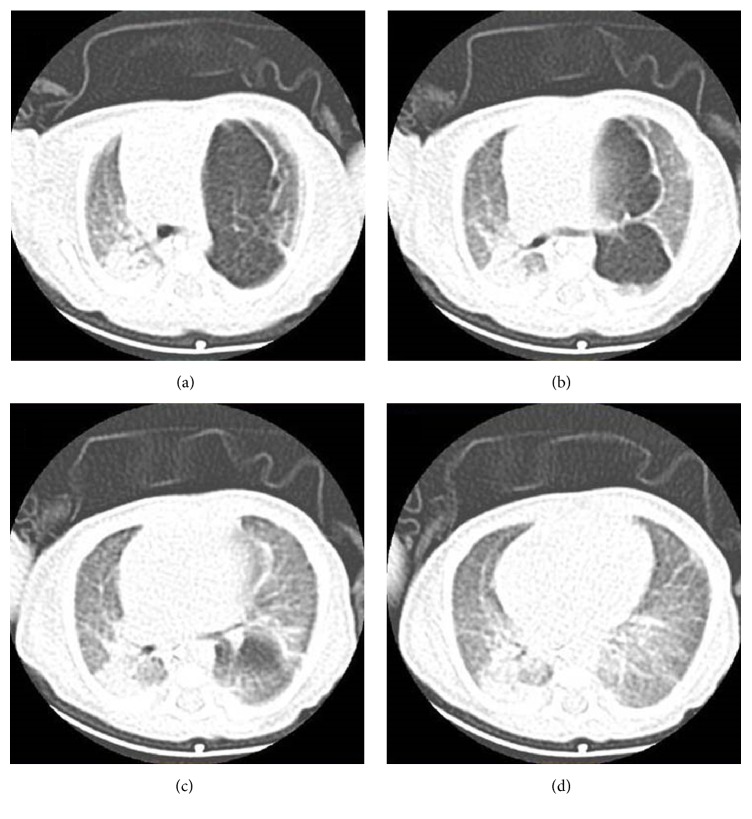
Computed tomography of the chest showing the presence of an emphysematous left upper lobe and atelectasis of the left lower lobe with subsequent contralateral mediastinal shift to the right causing atelectasis of the right lung.
